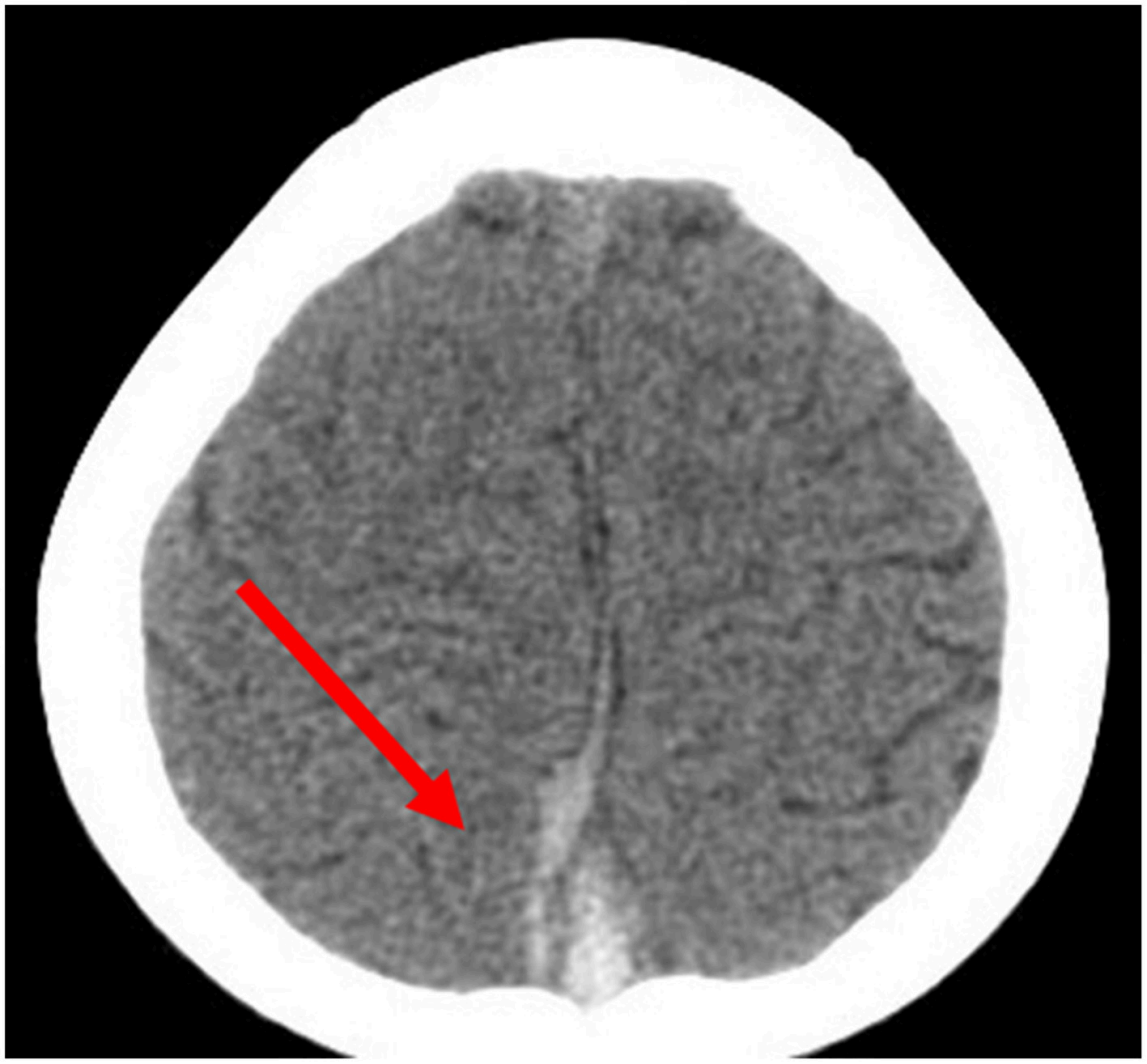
A CT scan before treatment showing a high-density shadow in the superior sagittal sinus.
